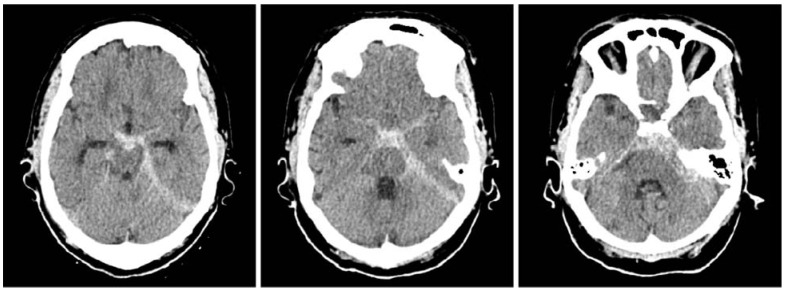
A 79-year-old woman with known basilar tip aneurysm presenting with acute onset of severe headache (same patient as in Figure 1). The non-contrast CT scan study demonstrates diffuse subarachnoid hemorrhage in the perimesencephalic cistern and left ambient cistern extending along the left tentorium, consistent with rupture of basilar tip aneurysm.
